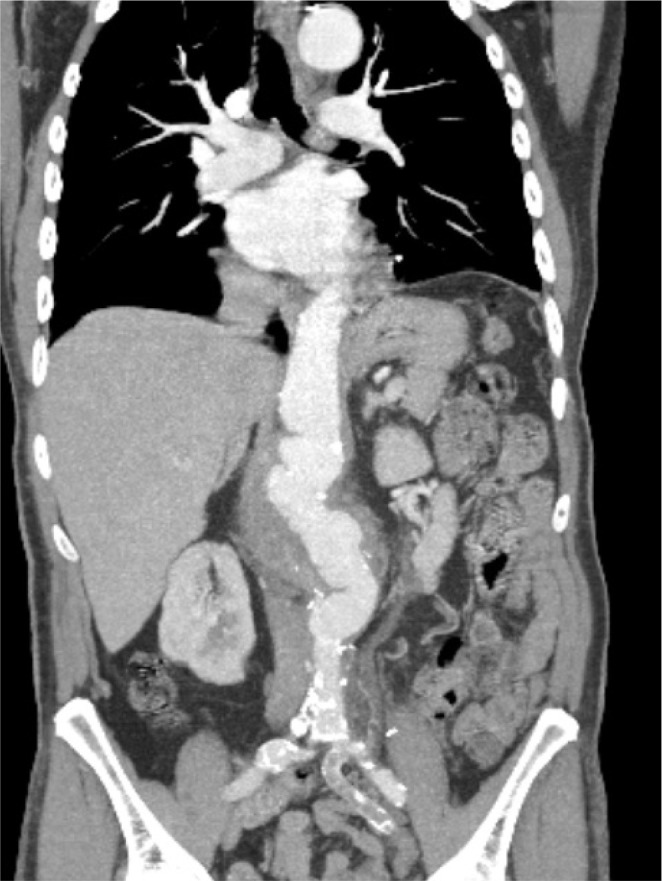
Preoperative computed tomography angiography of thoracoabdominal aneurysm
